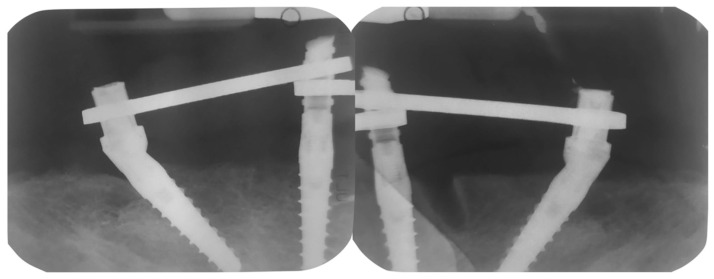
Implant X-rays immediately after load.
